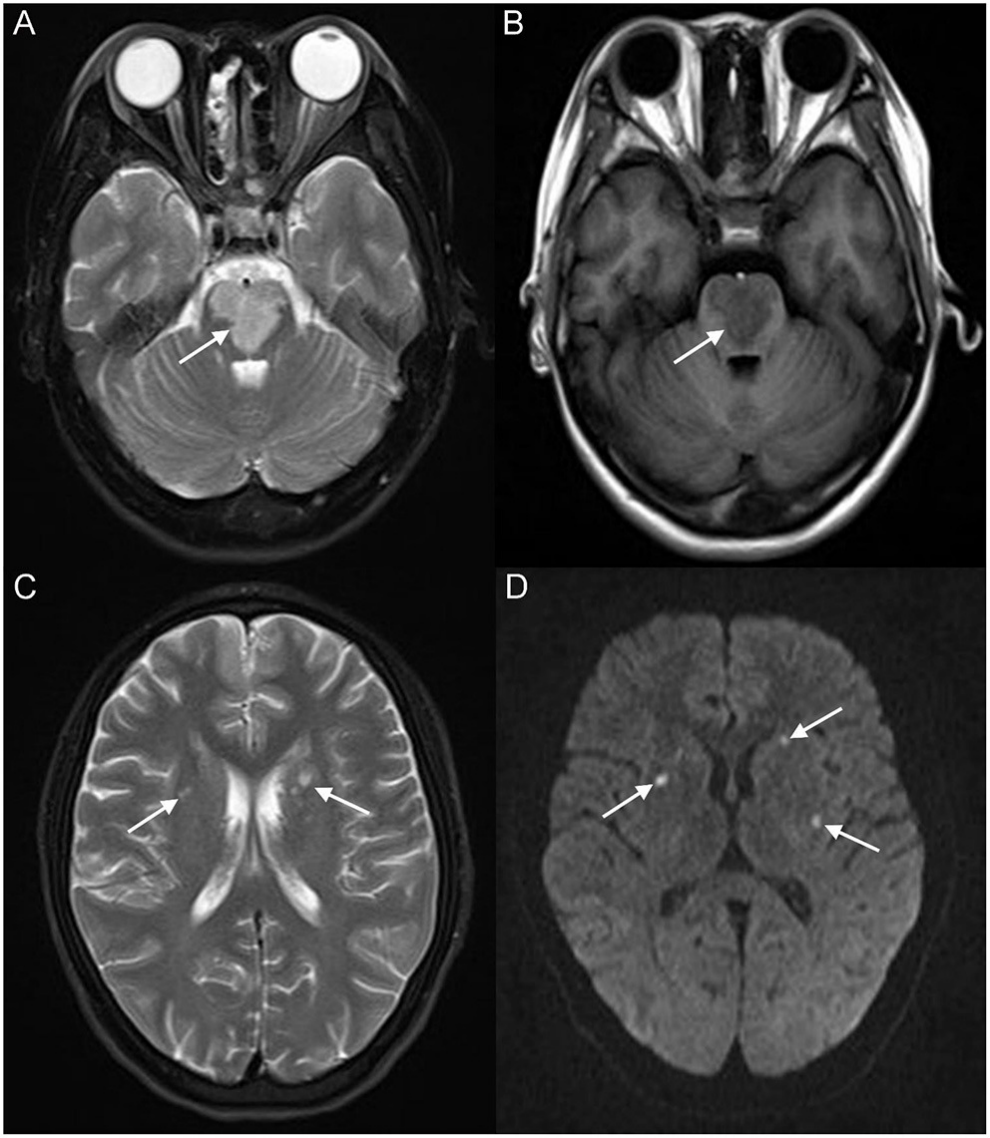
Brain magnetic resonance imaging (MRI) revealed extensive hyperintensity on T_2_-weighted imaging and hypointensity on T_1_-weighted imaging of the bilateral parts of the pons (white arrow) (A,B); a patchy hyperintensity on T_2_-weighted imaging of the bilateral basal ganglia, bilateral centrum semiovale (white arrow) (C), and right frontoparietal lobe, which presented a high signal on diffusion-weighted imaging (DWI) (white arrow) (D).
